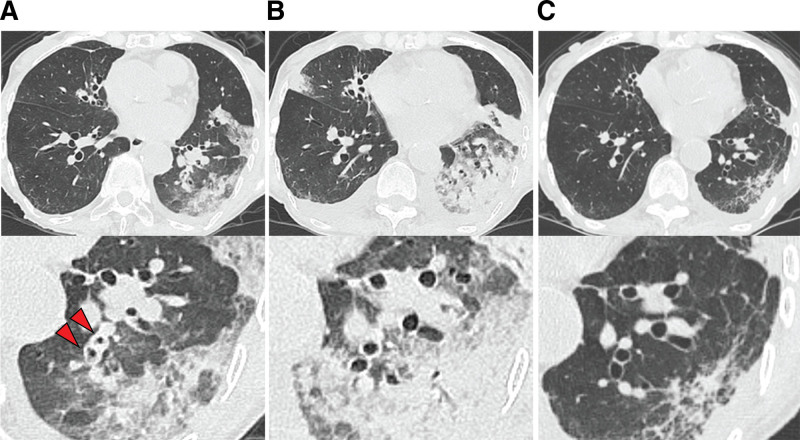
Chest CT on the day of admission (A) showed consolidations in the bilateral lower lobes and right middle lobe, along with bronchial wall thickening and mucus plugs (arrows indicate bronchial wall thickening). On hospital day 6 (B), consolidations in the bilateral lung fields had worsened. On hospital day 15 (C), after 9 days of treatment with prednisolone at 0.6 mg/kg/d, the consolidations had improved. CT = computed tomography.
